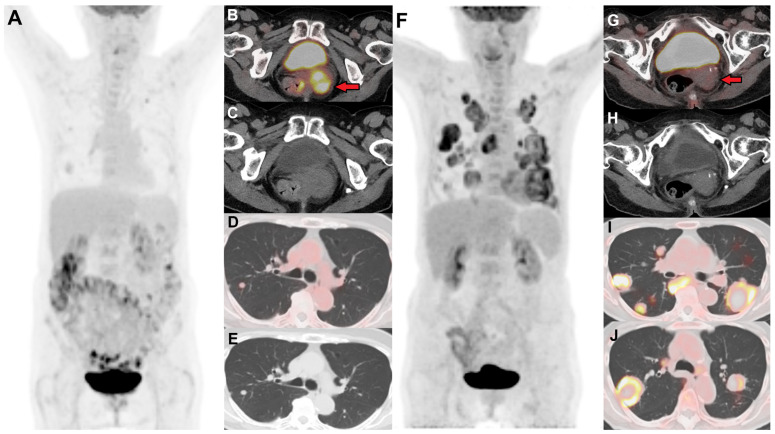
(A) Baseline FDG PET/CT in a female with stage IVB cervical cancer showing an FDG-avid primary cervical lesion ((B) (red arrow), (C)), pelvic nodes, and a few lung nodules suspicious for metastases (D,E). (F) Follow-up PET/CT 12 months post-chemoradiation shows resolution of FDG avidity in the cervix ((G) (red arrow), (H)), consistent with post-radiation treatment changes. However, there are new and increased sizes of numerous FDG-avid bilateral pulmonary metastases (I,J), with some lesions showing central necrosis.
